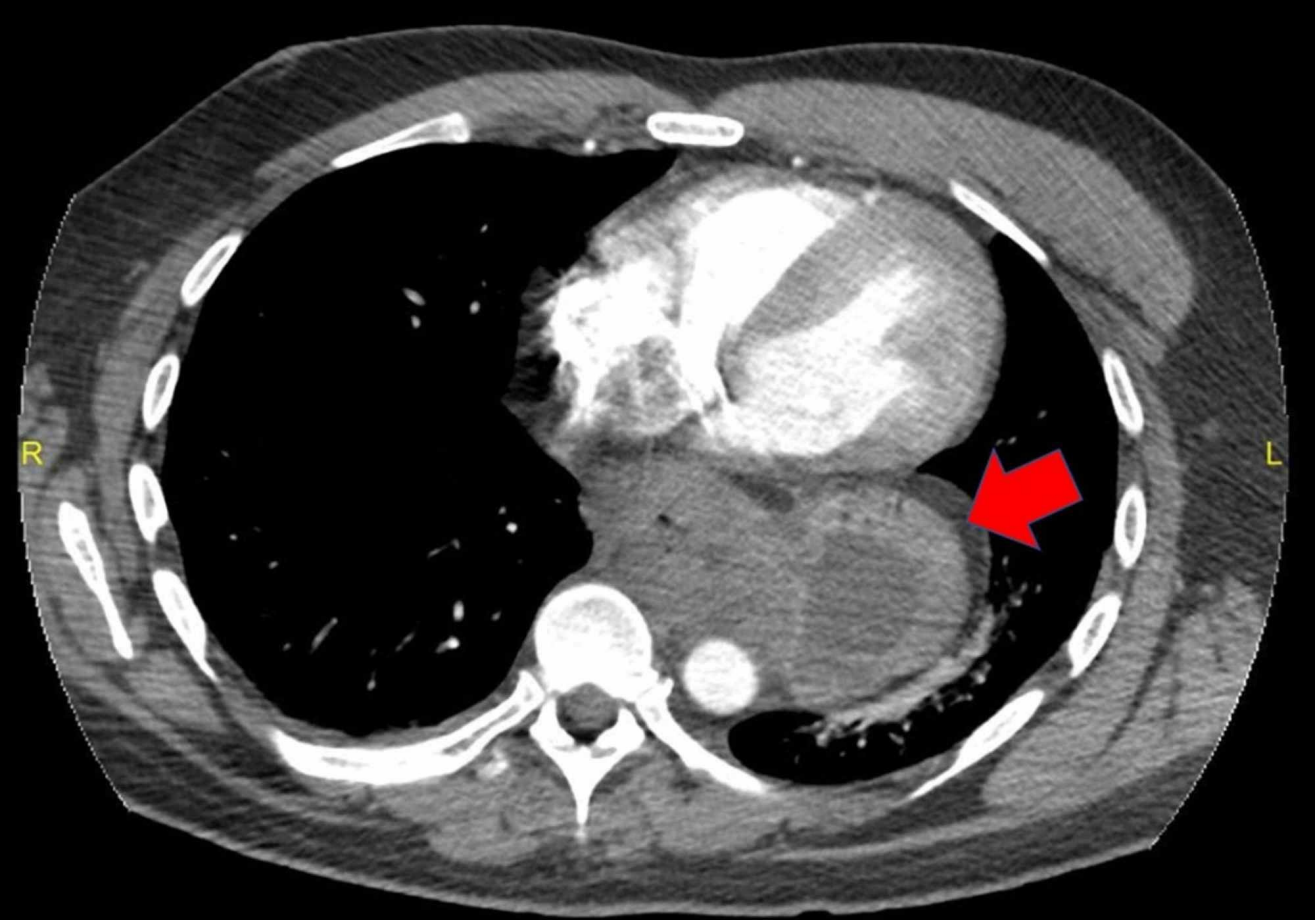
Contrast-enhanced computed tomography showed a large hiatal hernia with marked thickening of the distal esophagus and gastroesophageal junction
